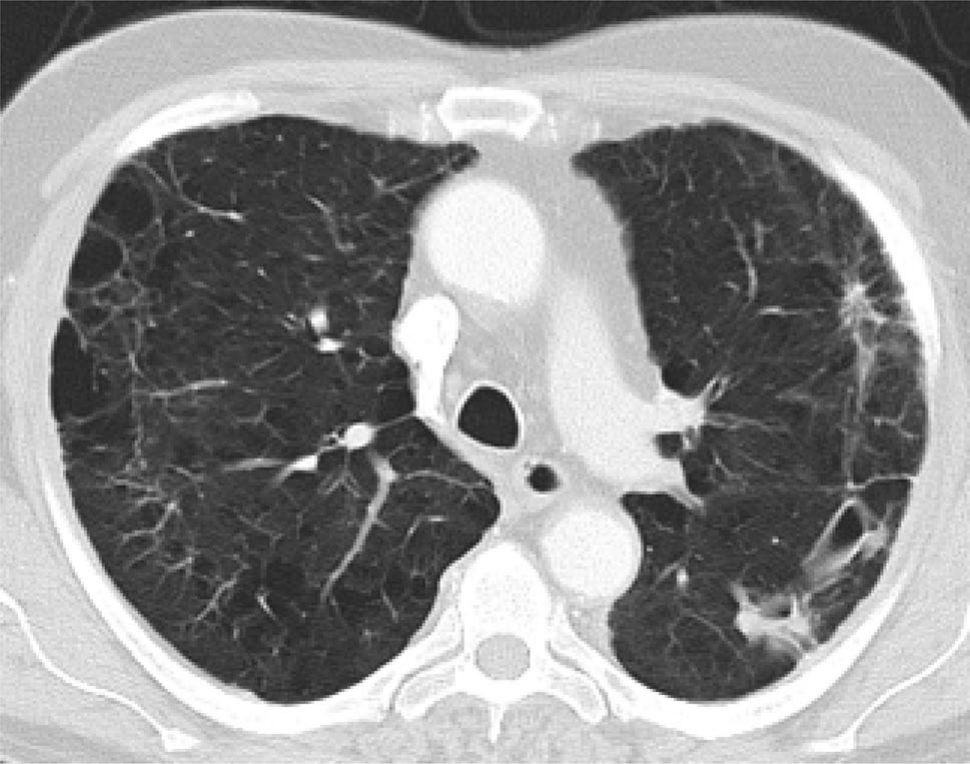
CT thorax of a long term smoker with COPD presenting with increasing shortness of breath for 20 years. CT scan shows multiple nodules on the left side, two of which had cavitated, in association with a raised *Aspergillus* IgG titre, all in keeping with chronic cavitary pulmonary aspergillosis
